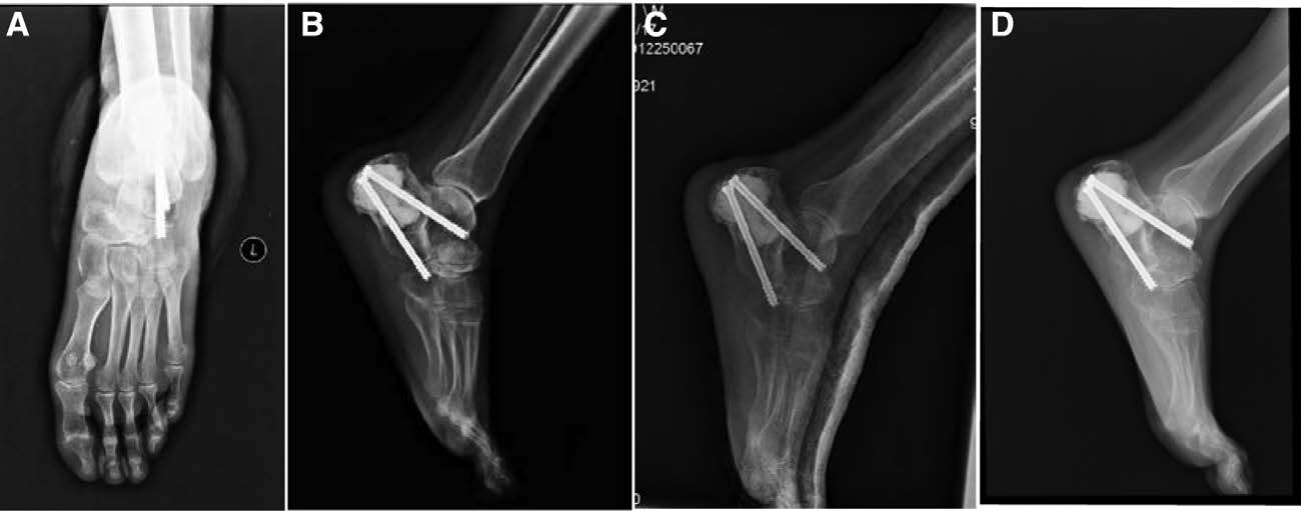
The arthrodesis healed successfully without fixation failure in 2 years of follow-up. (A, B) Anteroposterior and lateral X-ray images 7 days after surgery. (C) Lateral X-ray image 8 weeks after surgery. (D) Lateral X-ray image 8 months after surgery, the arthrodesis healed successfully.
